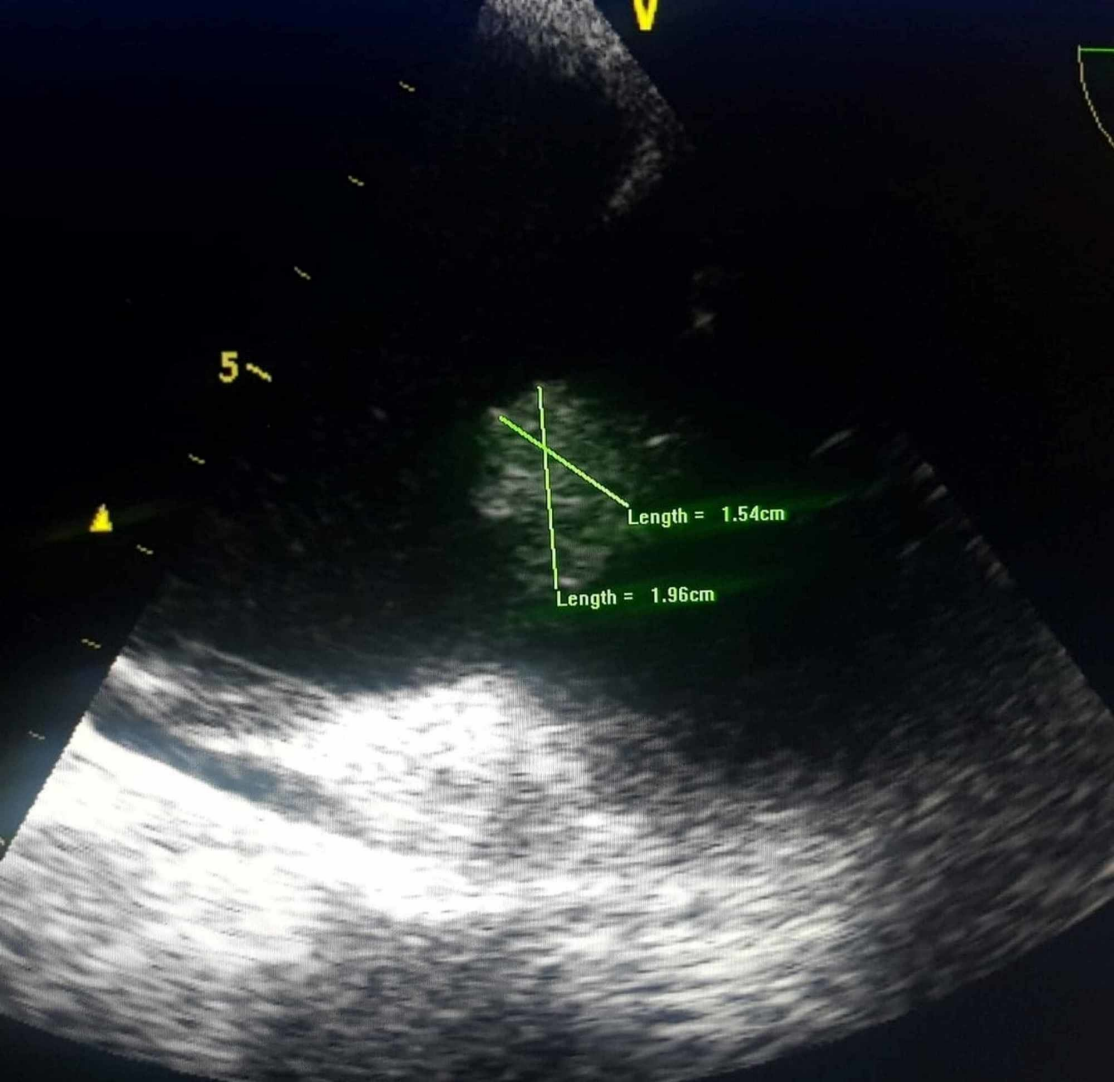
TTE showing 1.9 x 1.5 cm echo density attached to the tricuspid valve leaflet. TTE, transthoracic echocardiography
